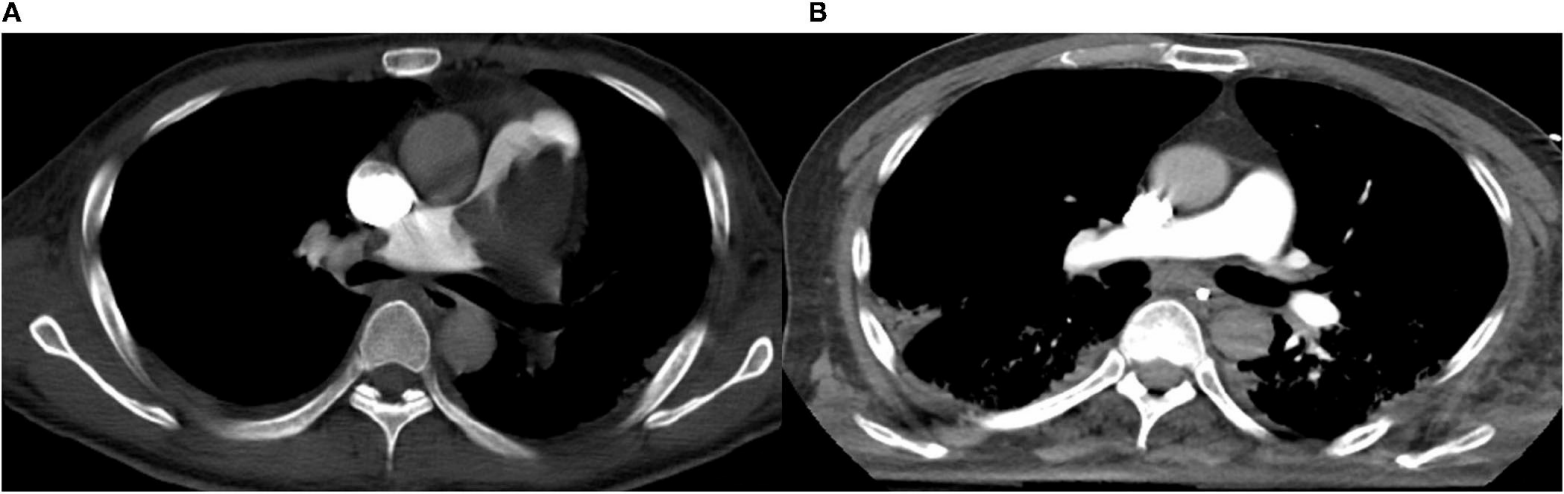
(A) Computed tomography pulmonary angiography (CTPA) demonstrates pulmonary embolism (PE). (B) Computed tomography pulmonary angiography (CTPA) demonstrates that residual thrombus markedly improved.
